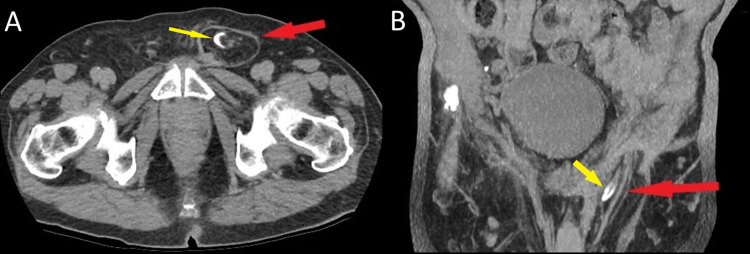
Preoperative CT scan of the abdomen and pelvis (A, B) Recurrence of left inguinal hernia (red arrow) containing ureter (yellow arrow)
